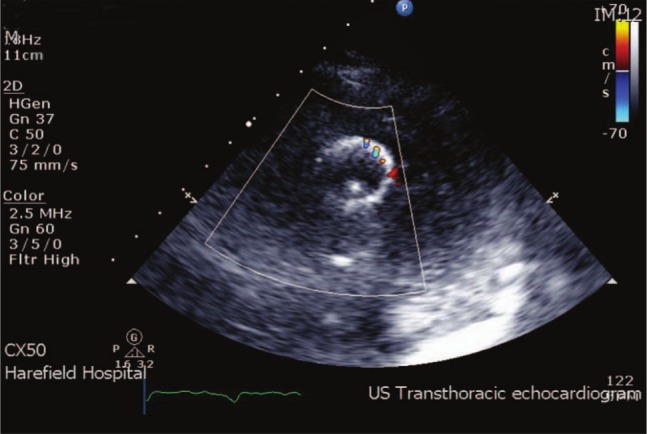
Echocardiographic assessment during second admission: image suspicious for pump thrombus.
